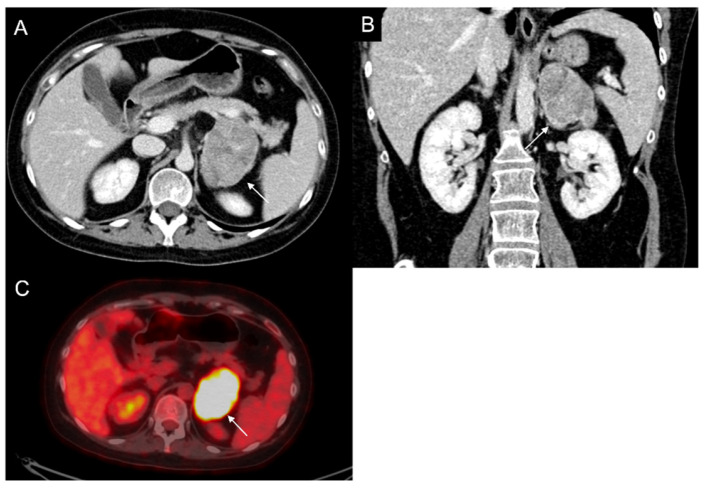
60-year-old woman with history of breast cancer and adrenal metastasis. Left adrenal mass with inhomogeneous enhancement (arrow) on CT portal phase in axial (A) and coronal (B) planes, and positive PET (C).
